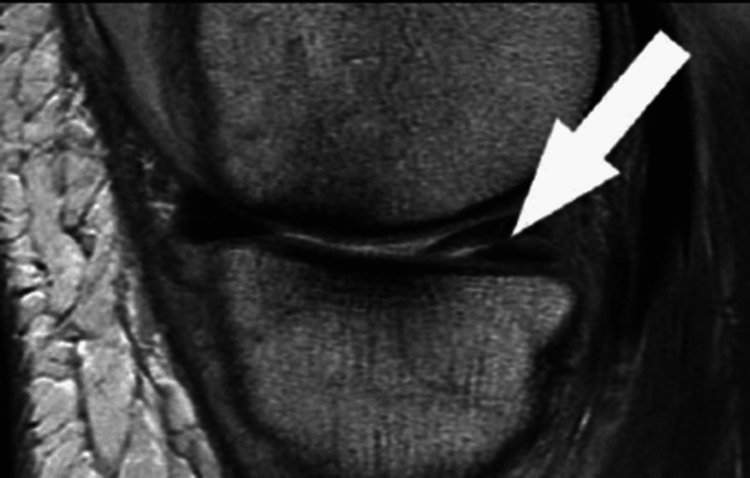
Sagittal MRI of posterior horn medial meniscus horizontal tear Note. Image from Bhan K (2020) [2] MRI: magnetic resonance imaging
